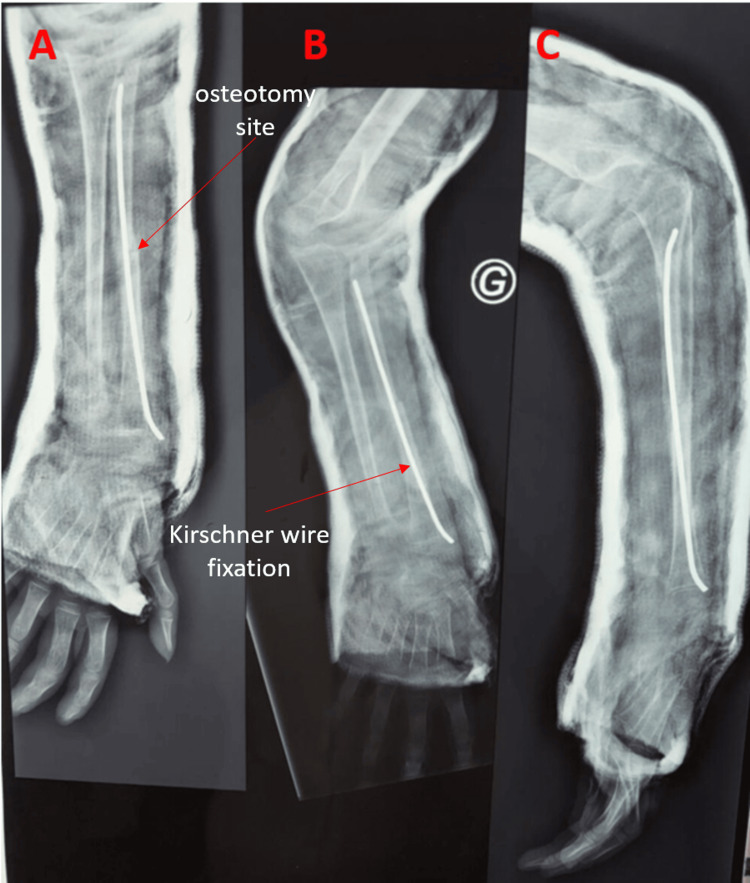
Postoperative radiographs after radial derotational osteotomy of the left forearm. (A) Anteroposterior view showing radial osteotomy fixed with a Kirschner wire.
